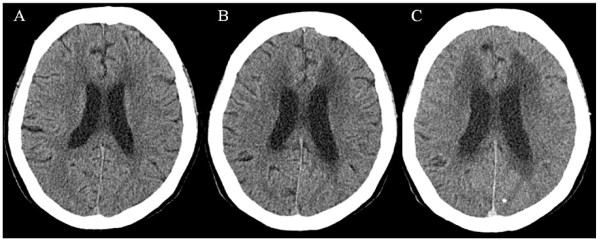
Cranial computed tomography images (A) one month before admission, (B) on admission, and (C) three weeks after admission. No mass lesions were present. However, mild hydrocephalus exacerbation with disappearing sulcus and ventricular distention were observed.
